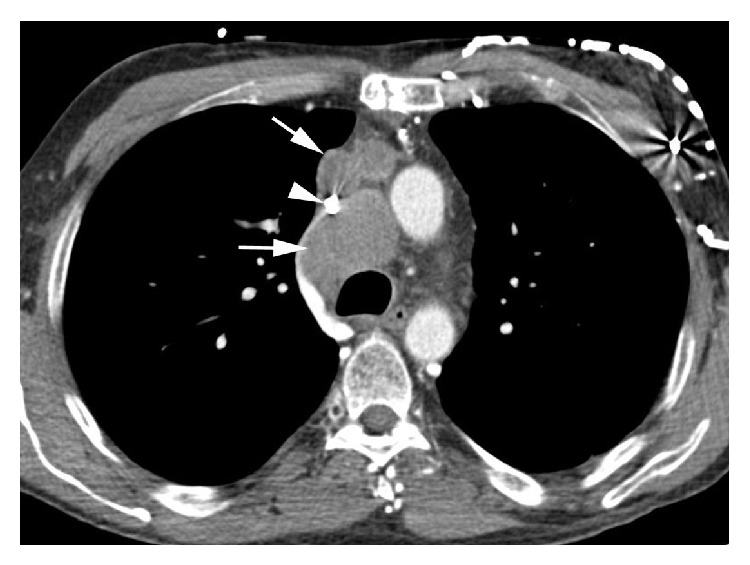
Axial IV contrast-enhanced CT image shows marked right paratracheal and anterior mediastinal lymphadenopathy (arrow) with marked compression of the superior vena cava (arrowhead).
